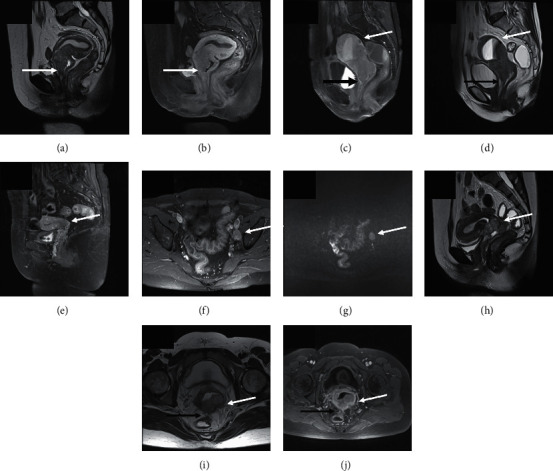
(a, b) A 53-year-old woman with IIA cervical cancer: T2-weighted sagittal image (a) and T1-weighted contrast-enhanced sagittal image (b) show a cervical mass with infiltration of the upper vagina (white arrow). (c, d) A 60-year-old woman with IIA cervical cancer: T1-weighted contrast-enhanced sagittal image (c) and T2-weighted sagittal image (d) show a bulky cervical mass with vaginal extension (black arrow) as well as uterine body invasion (white arrow). (e–g) A 67-year-old woman with IIIC cervical cancer: T2-weighted sagittal image (e) shows a cervical mass (white arrow); axial contrast-enhanced image (f) and diffusion-weighted image (g) show an enlarged pelvic lymph node (white arrow) with moderate enhancement and diffusion restriction, indicating pelvic lymph node involvement. (h–j) A 49-year-old woman with IVA cervical cancer: T2-weighted sagittal image (h) shows a cervical mass (white arrow); axial T2-weighted image (i) and contrast-enhanced image (j) show the cervix tumor (white arrow) with rectal wall invasion (black arrow).
